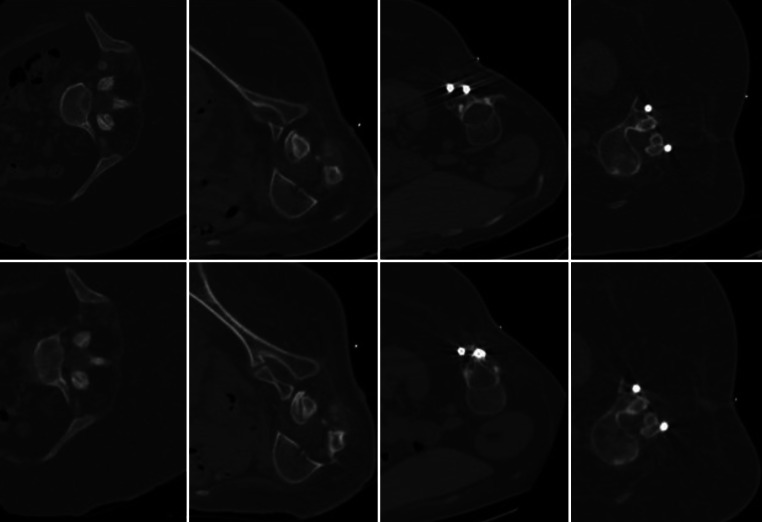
Standard dose (SD; *upper row*) and low dose (LD; *lower row*) multi-detector computed tomography (MDCT) scans for procedure planning of intrathecal nusinersen administration in four patient cases with spinal muscular atrophy (SMA). Tube current was reduced from 133 mA in SD scans to 20, 40, 67 and 27 mA, in LD scans, resulting in considerable dose reductions. The SD scans were reconstructed using hybrid iterative reconstruction (iDose^4^; Philips Healthcare, Best, The Netherlands) while the LD scans were reconstructed using model-based iterative reconstruction (IMR; Philips Healthcare, Best, The Netherlands). The LD images demonstrate a blurrier appearance, but the reduced image quality of the LD scans did not impair the confidence for intervention planning. Due to the model-based iterative reconstruction, the LD images show less streak artifacts from metal implants (third and fourth column)
